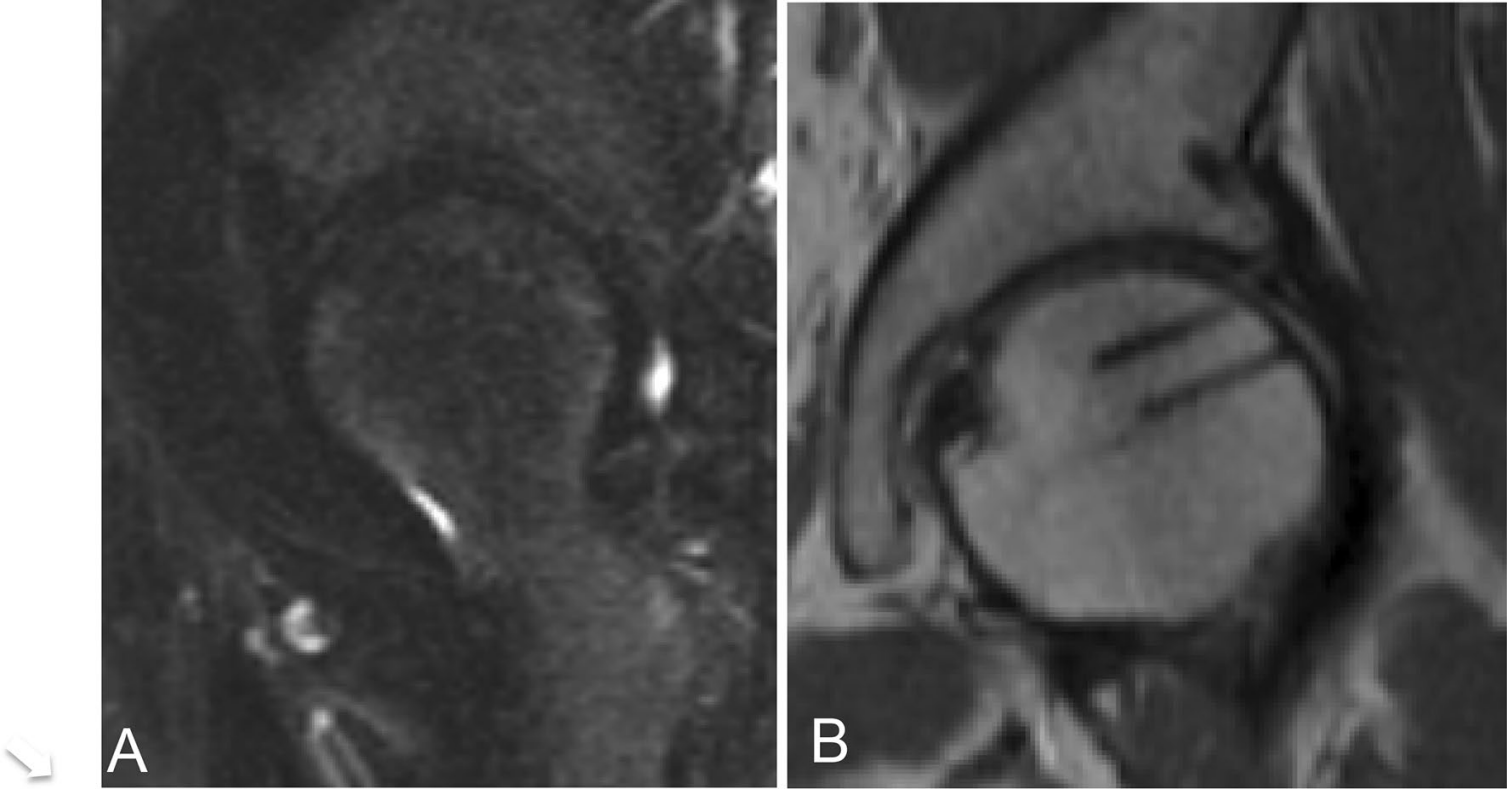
MRI findings a oblique sagittal T2-weighted fat saturation MR views showed complete disappearance of bone oedema pattern at 1 year after surgery. b Coronal proton density weighted MR view shows disappearance of fracture line and low-intensity bars indicating HA/PLLA threaded pins (arrows)
